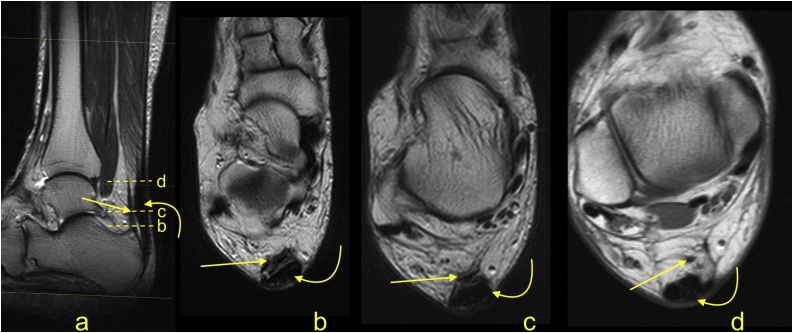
The plantaris tendon (straight arrow) contributes to the paratenon. A 48-year-old patient with pain in the Achilles tendon. Distally, the plantaris tendon (straight arrow) divides into small parts and merges with the paratenon, mimicking fibrosis on the axial section. The insertion is the calcaneus (straight arrow on figure a). The MRI (PD-weighted) revealed thickening of the Achilles tendon (curved arrow).
